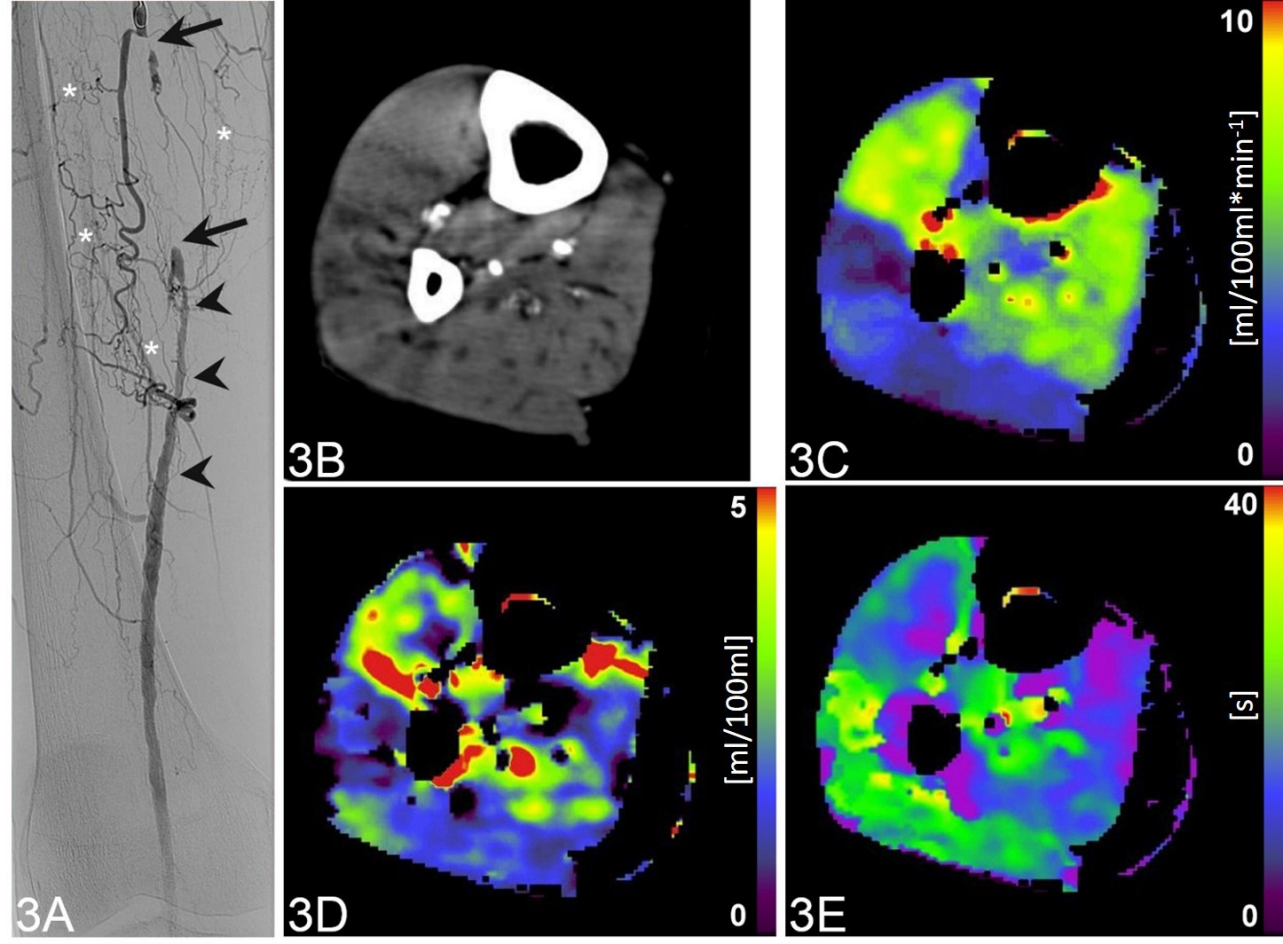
Occlusion of the right-sided superficial femoral artery of a 72-year-old patient. Coronal digital subtraction angiography image (A) shows a complete intermediate-length occlusion (arrows) of the right right-sided superficial femoral artery, which is extensively collateralized (asterisks). The distal segment of the vessel is patent, however, with caliber irregularities (arrowheads). CTP of the lower leg (B, maximum intensity projection CT image during 60s after contrast administration) with color-coded parametric maps (C-E) reveals a mean blood flow of 5.54 ml/100ml*min^-1^ (C), mean blood volume of 0.81 ml/100ml (D) and mean transit time of 12.85 s (E).
